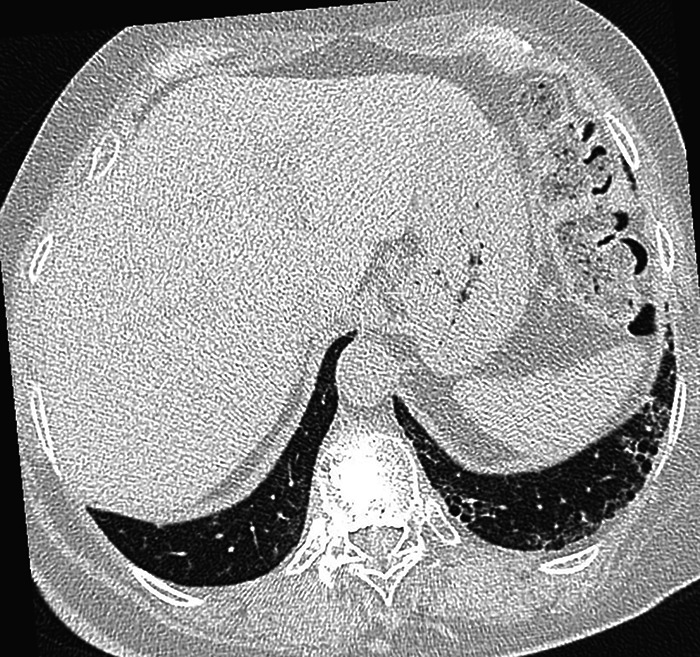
Axial initial CT image of lungs showing bi-basal cystic images, especially on the left and reminiscent of honeycomb, suggestive of early pulmonary fibrosis.
